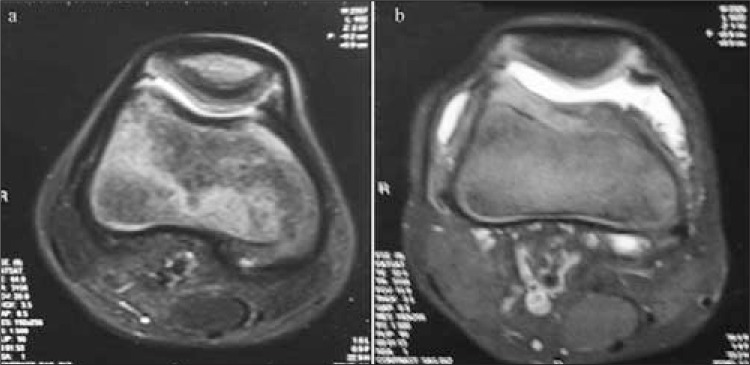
The axial fat-saturated T2 weighted MRI images before radiosynovectomyshow only minimal suprapatellar effusion (a), synovial hypertrophy,significant suprapatellar effusion and more than 50% cartilage loss 6months after radiosynovectomy (b)
